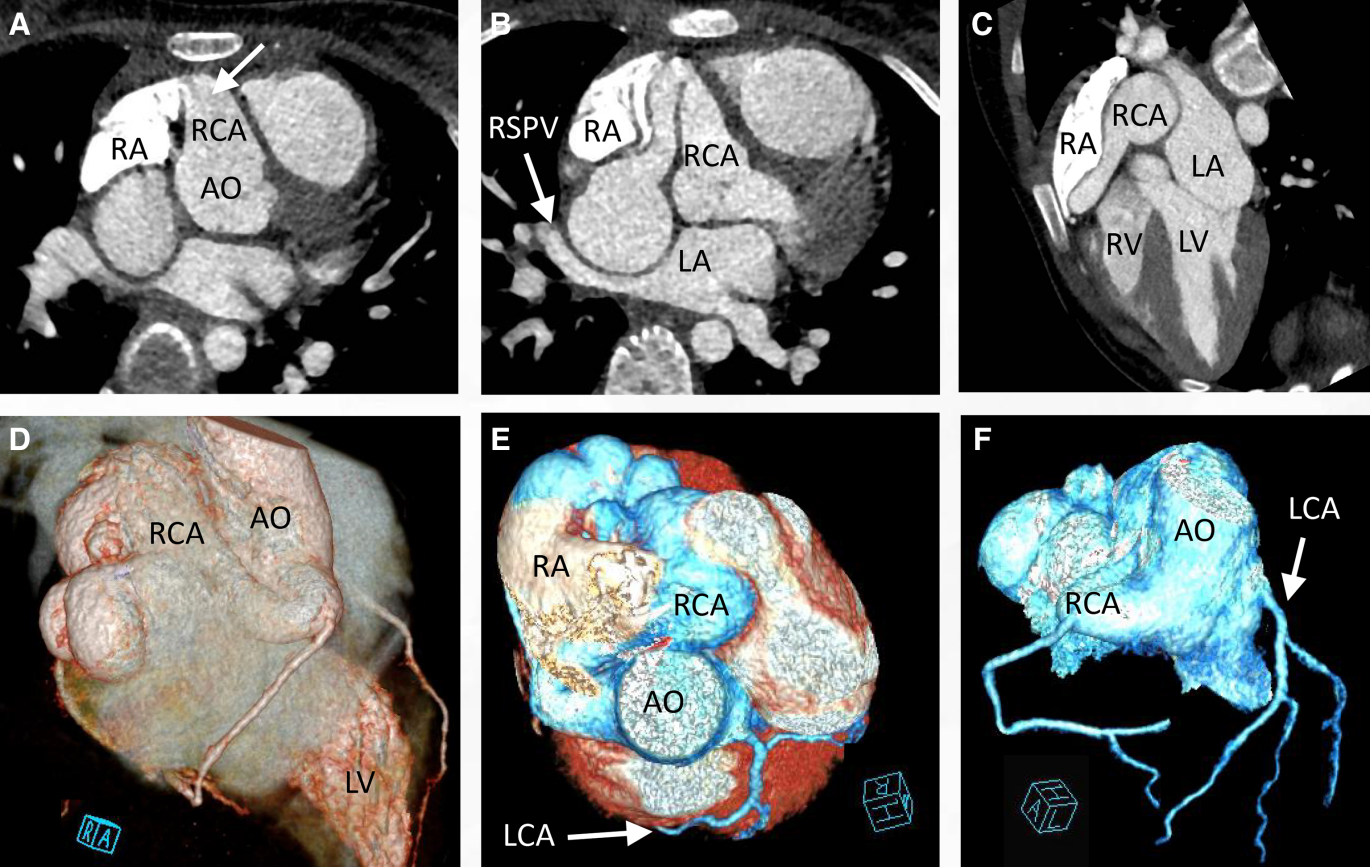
Cardiac computed tomography angiography preoperatively confirmed that the right coronary artery was significantly enlarged (A, arrow) to form a pseudoaneurysm (B), with the right superior pulmonary vein remarkably compressed (B, C, arrow). Three-dimensional reconstruction of the cardiac CTA indicated that the enlarged right coronary artery was tortuous (D, E) and the left coronary artery functioned normally (F, arrow). CTA, computed tomography angiography.
